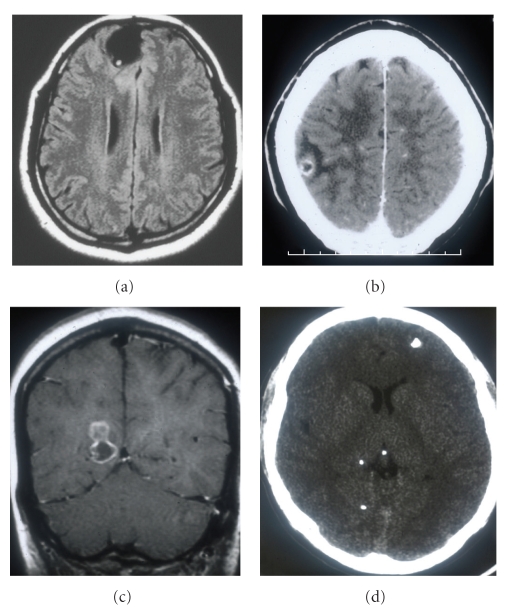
(a) MRI image revealing vesicular cysticerci (the central dot represents the scolex). (b) Cyst beginning to degenerate with perilesional edema and enhancement in the colloidal stage. (c) Cyst in the granular stage without perilesional edema. (d) CAT scan revealing multiple calcifications in the calcified stage.
